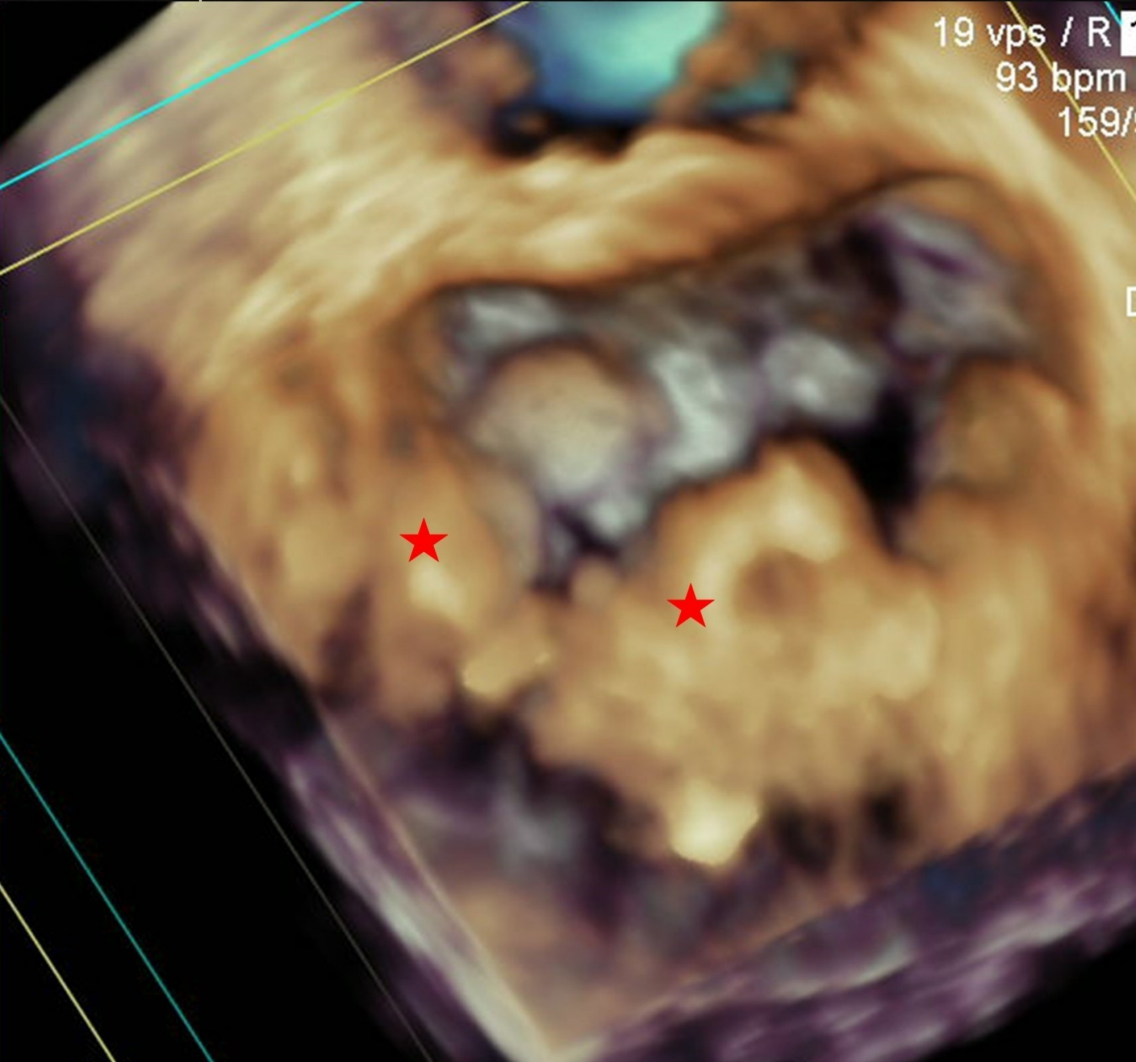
Three-dimensional transesophageal echocardiography. En face view of mitral valve showed two discrete nonmobile calcified masses (red asterisks), involving at least 50% of the posterior mitral valve annulus, with central areas of echolucency resembling liquefaction necrosis.
